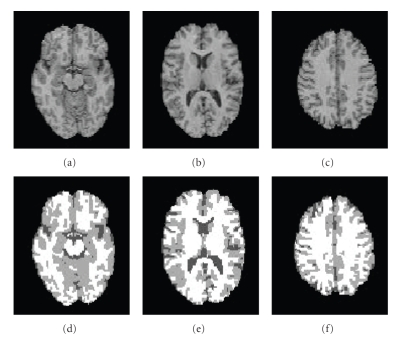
Examples of human brain MRI cross-sectional images. (a), (b), (c): Original MRI cross-sectional human brain images. (d), (e), (f): Unsupervised three-dimensional Markovian segmentations.
